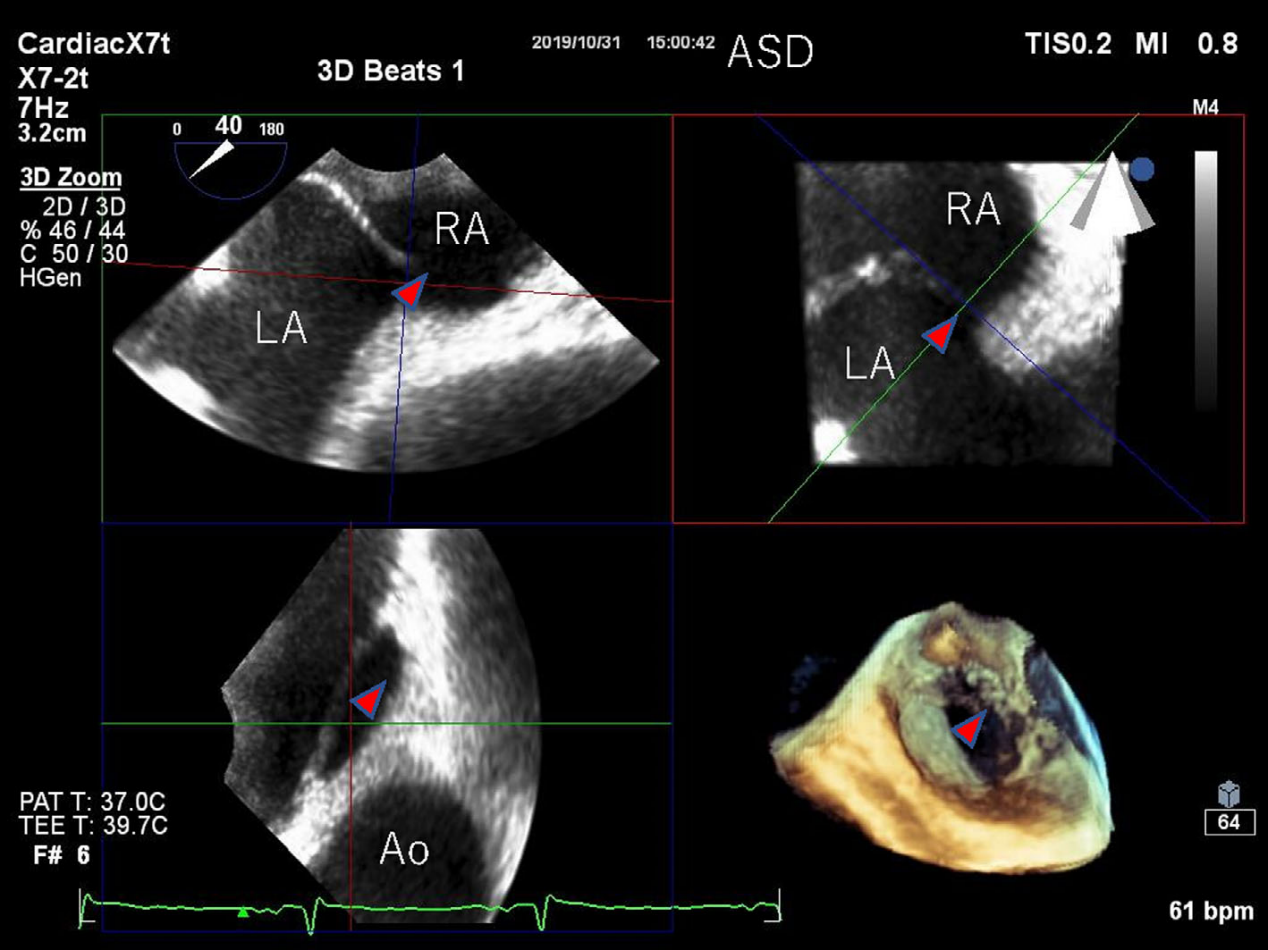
Trans‐oesophageal echocardiography in the sitting position. Ao, aorta; ASD, atrial septal defect (red arrow); LA, left atrium; RA, right atrium
